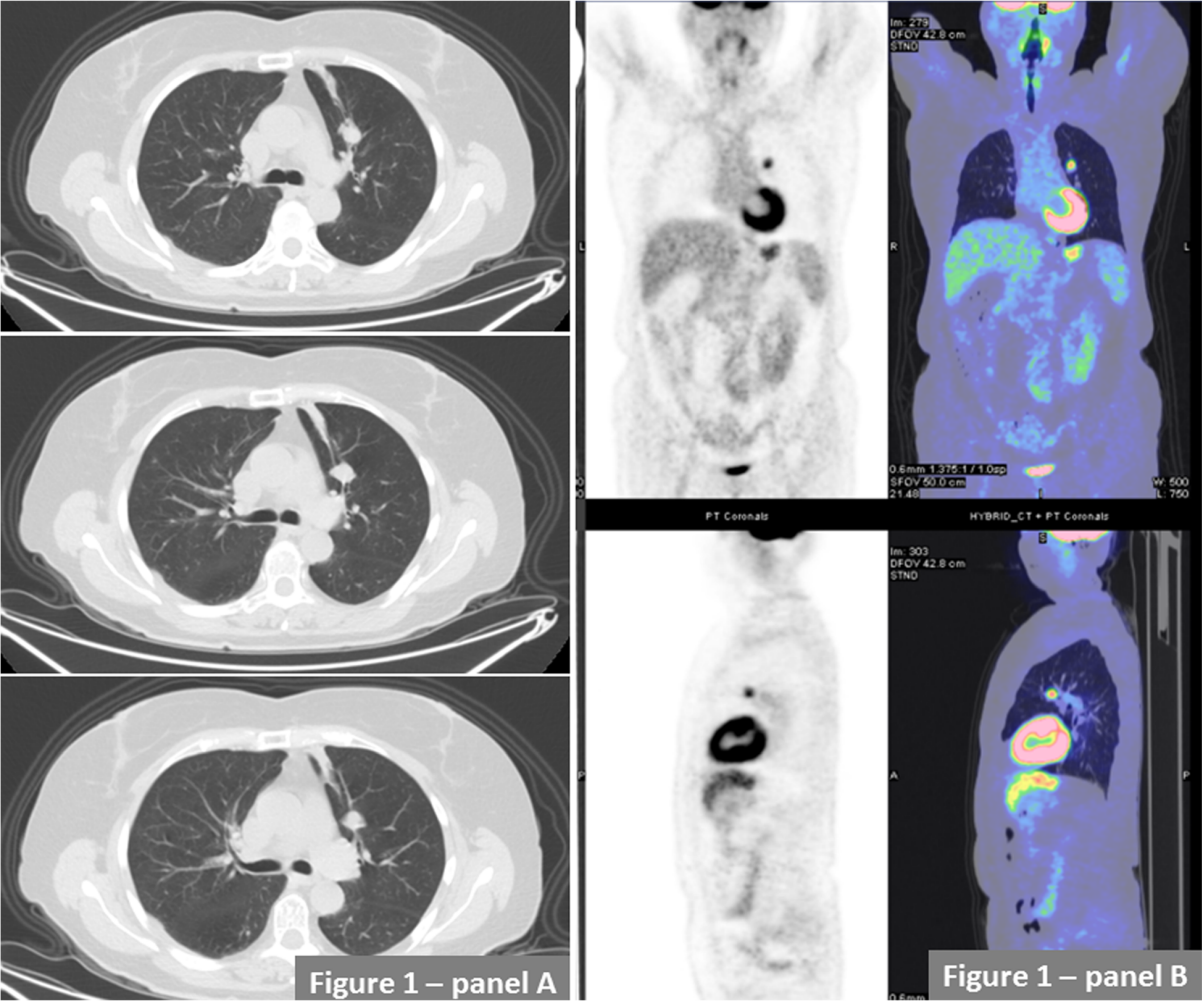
Panel a. Chest computed tomography (CT) scan (panel a) showing a single nodular opacity of 1.6 × 1.3 cm in the anterior segment of the left upper lobe. Panel b. Fluorodeoxyglucose positron emission tomography/computed tomography (FDG PET/CT) showing a nodular area of increased metabolic rate located in the same area of the CT-identified lung opacity
